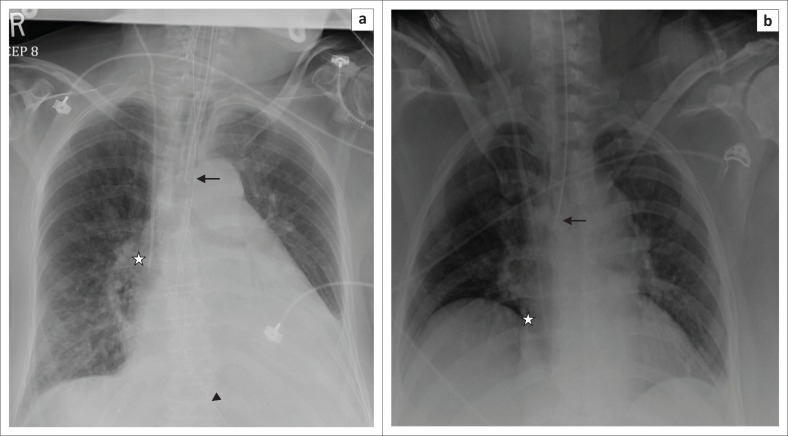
(a) Magnified chest radiograph showing an accurately placed endotracheal tube with its distal end (arrow) located above the carina. Other lines and tubes visualised are the right internal jugular vein central venous catheter (star) and the nasogastric tube (arrow head); (b) chest radiograph showing a malpositioned endotracheal tube with its tip in the right main bronchus (arrow). A central venous catheter is noted in the right atrium (star).
